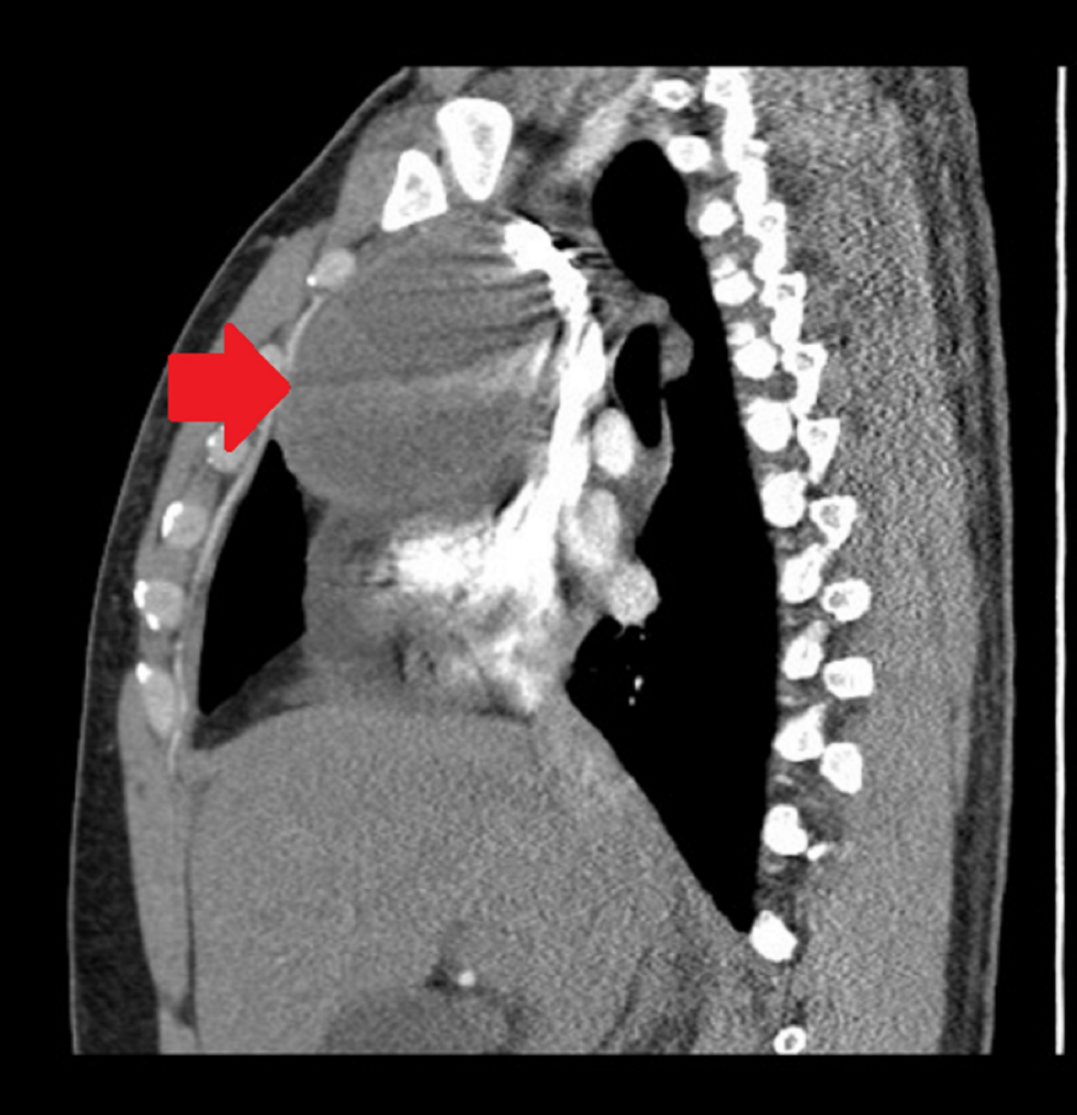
Computed tomography of the chest showing anterior mediastinal mass
